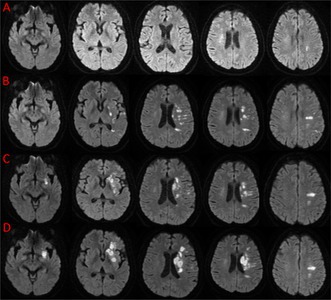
FIGURE 2 Panels A–D show axial diffusion‐weighted MRI sequences from patient 2 over time, demonstrating the evolution of ischemic lesions in the brain at 5 anatomical levels (from left to right): the mesencephalic level, basal ganglia level, level above.
